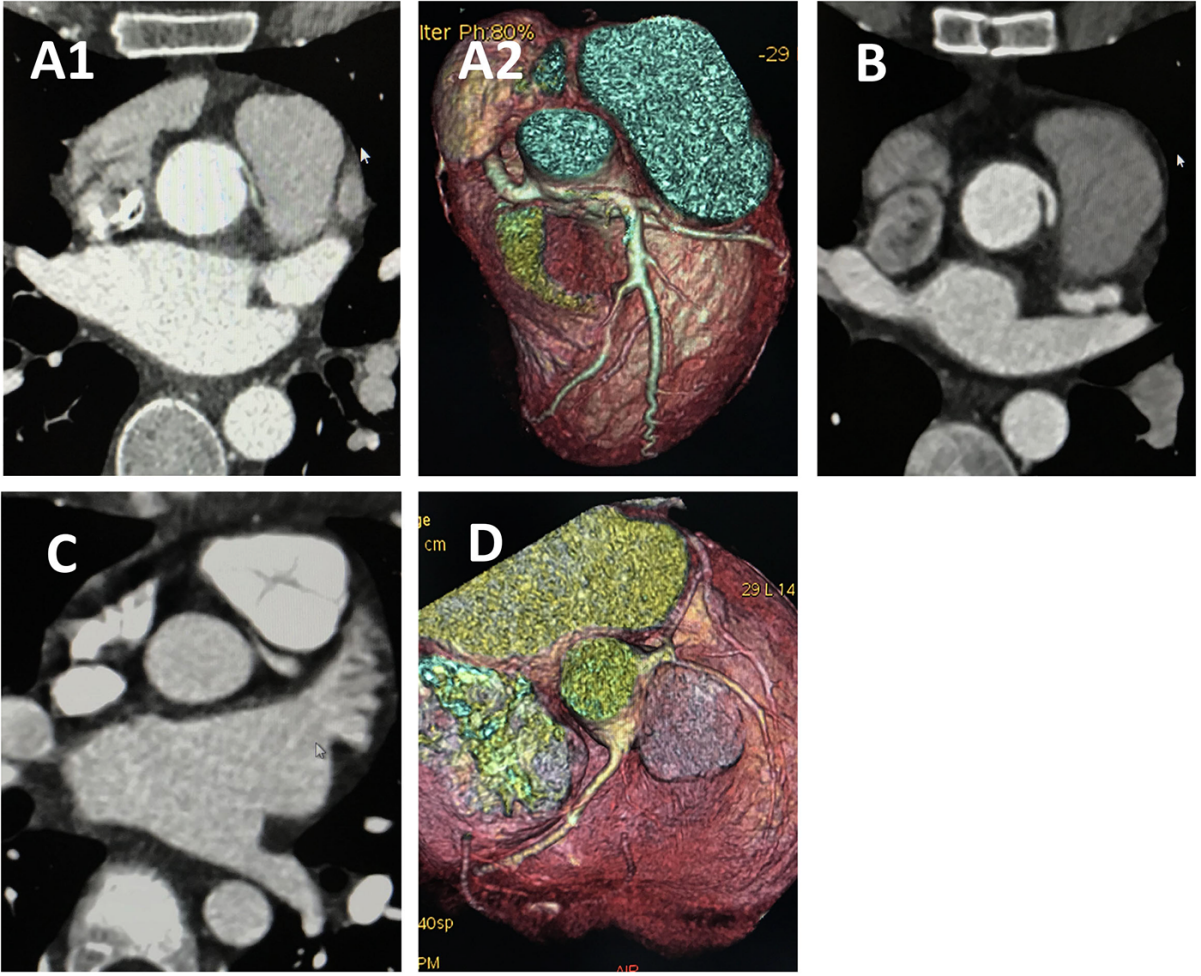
CCTA of case 7 and case 4 underwent angioplasty. Case 7 underwent angioplasty with pulmonary artery patch: (A) Preoperative images showing anomalous left coronary artery arising from the right sinus. (B) Follow-up images showing proximal left main coronary artery without visible stenosis. Case 4 underwent angioplasty with pericardial patch: (C) Preoperative images showing anomalous left coronary artery arising from the right sinus. (D) Follow-up images showing proximal left main coronary artery without visible stenosis. CCTA, coronary computed tomography angiography.
